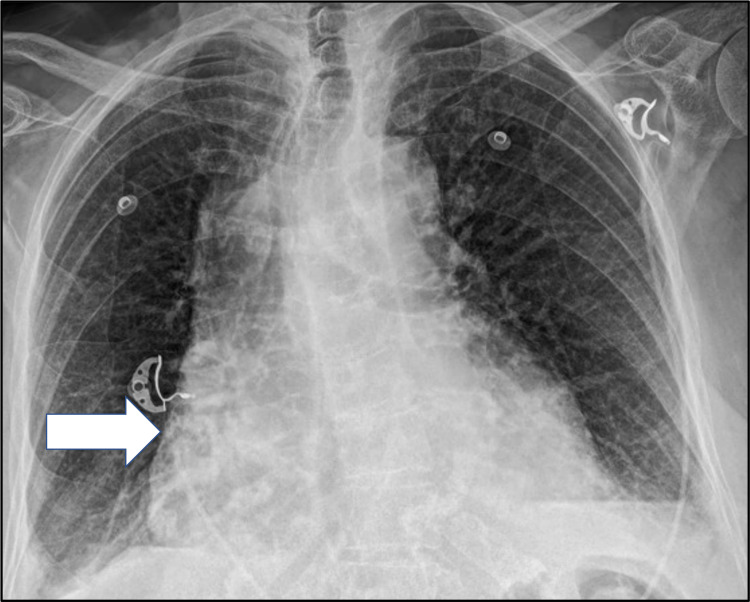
Chest x-ray showing cardiomegaly (white arrow)
